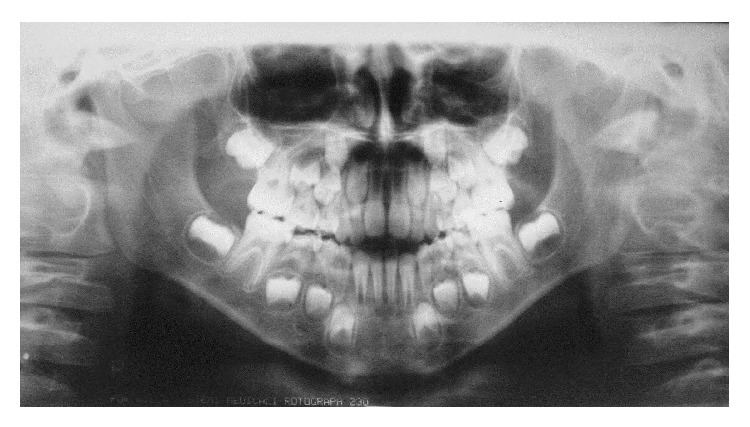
Panoramic radiograph. Normal TMJ structures, without injury.
